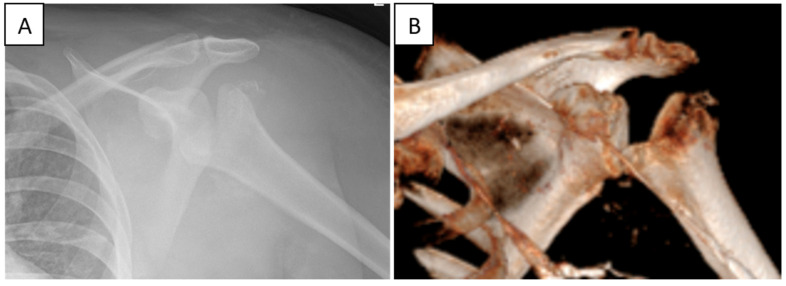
Radiographic and 3D imaging of a neurogenic (Charcot) shoulder joint in a patient with syringomyelia. (A) Plain radiograph showing marked destruction of the humeral head, joint subluxation, and loss of normal articular contours. (B) Three-dimensional reconstructed CT image highlighting severe bony fragmentation, deformity, and intra-articular debris consistent with advanced neuropathic arthropathy.
